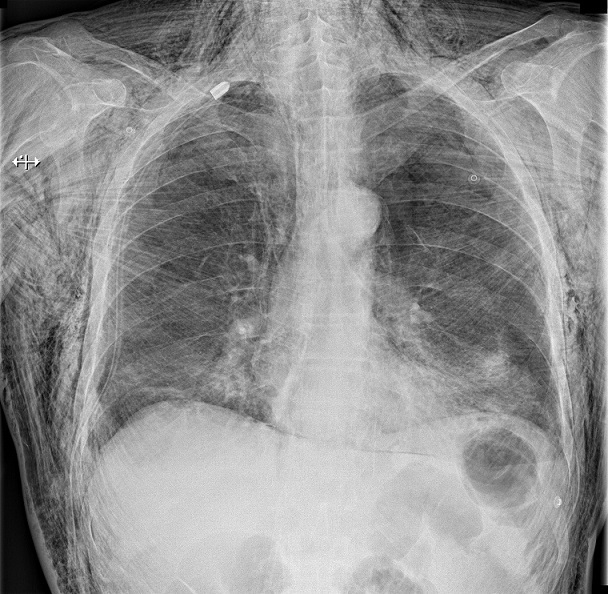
Chest x-ray showing a massive subcutaneous emphysema. Right lung reexpansion after chest tube placement
